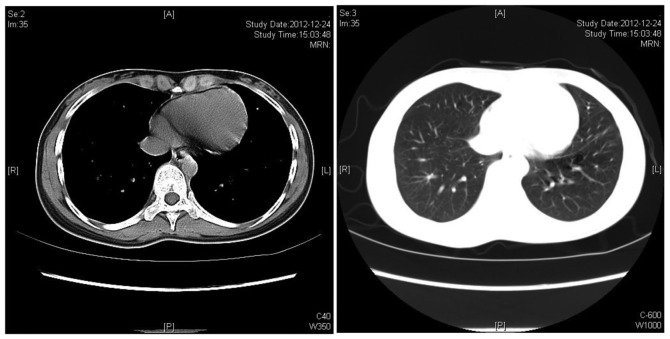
Computed tomography findings following 6 cycles of chemotherapy.
